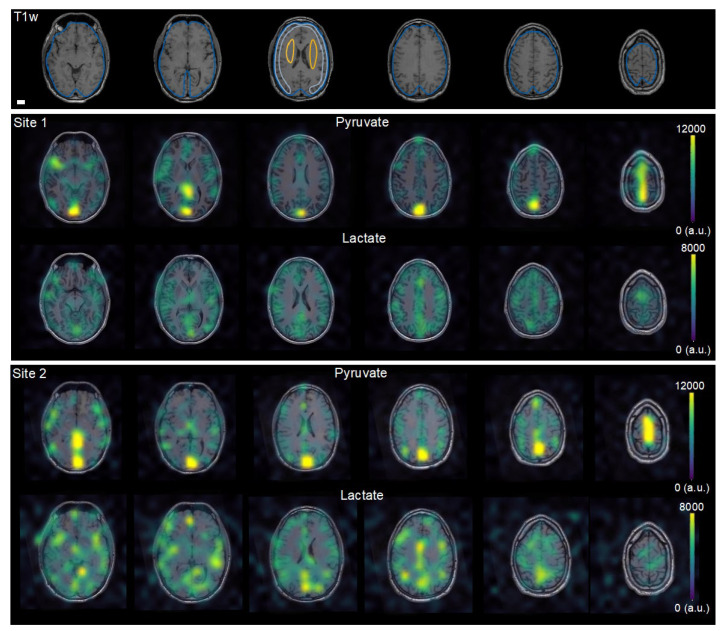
Comparison of hyperpolarized [1-^13^C]pyruvate MRI data from each site in the same participant. After injection of hyperpolarized [1-^13^C]pyruvate, dynamic images of [1-^13^C]pyruvate and [1-^13^C]lactate and were acquired in the same subjects at two sites. The six most cranial summed images are shown overlaid on T1 weighted (T1w) images from site 2, highlighting comparability between sites. Example regions of interest are shown for the whole brain (blue), deep white matter (yellow), and cortex (grey). The white scale equals 2 cm.
